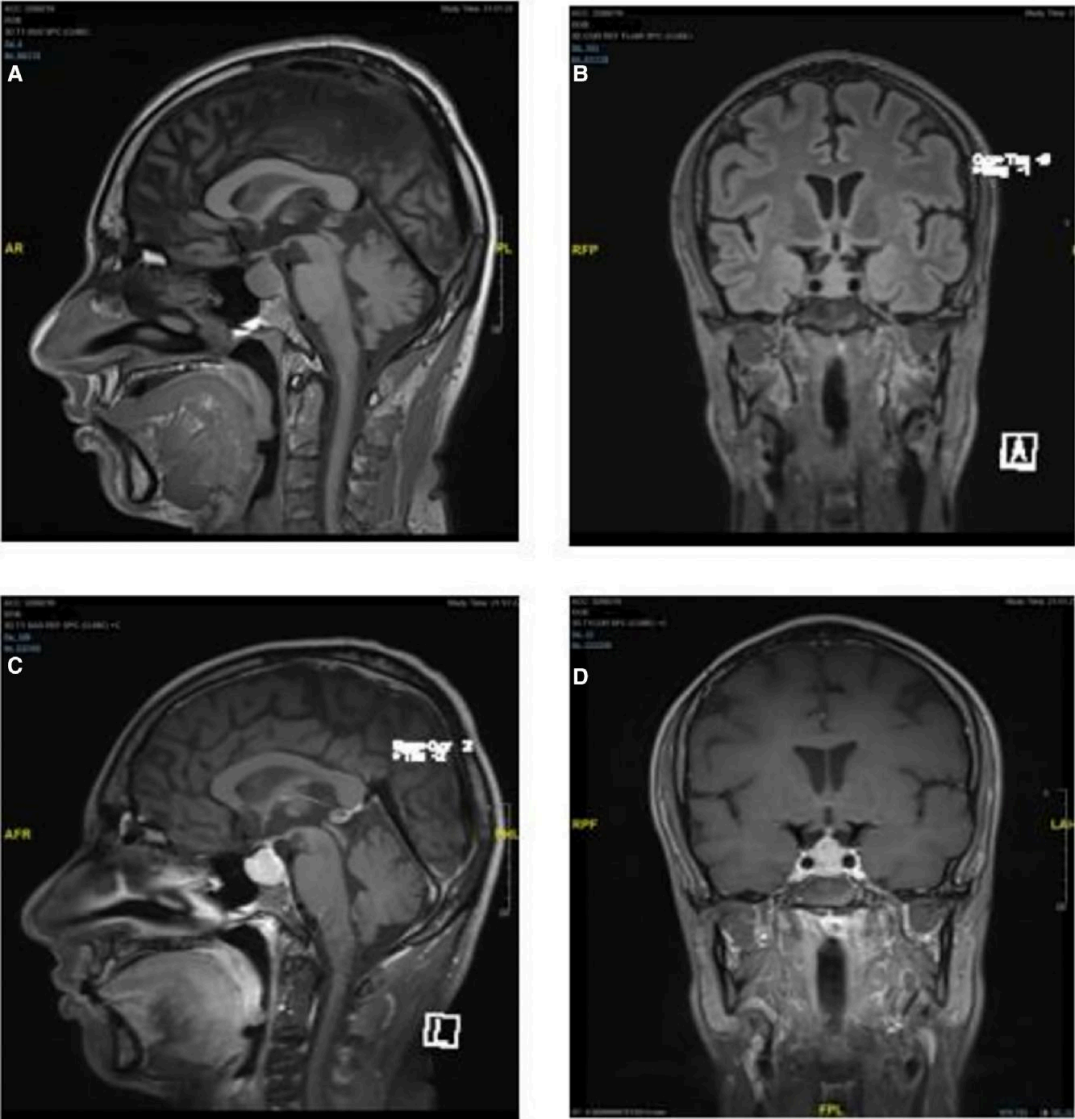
Initial pituitary magnetic resonance imaging scan displaying diffuse pituitary gland enlargement. (A) and (B) Unenhanced sagittal and coronal images indicated an enlarged pituitary gland with no compression on the optic chiasm. (C) and (D) Homogeneously enhanced sagittal and coronal images of the enlarged pituitary gland after Gd-DTPA.
